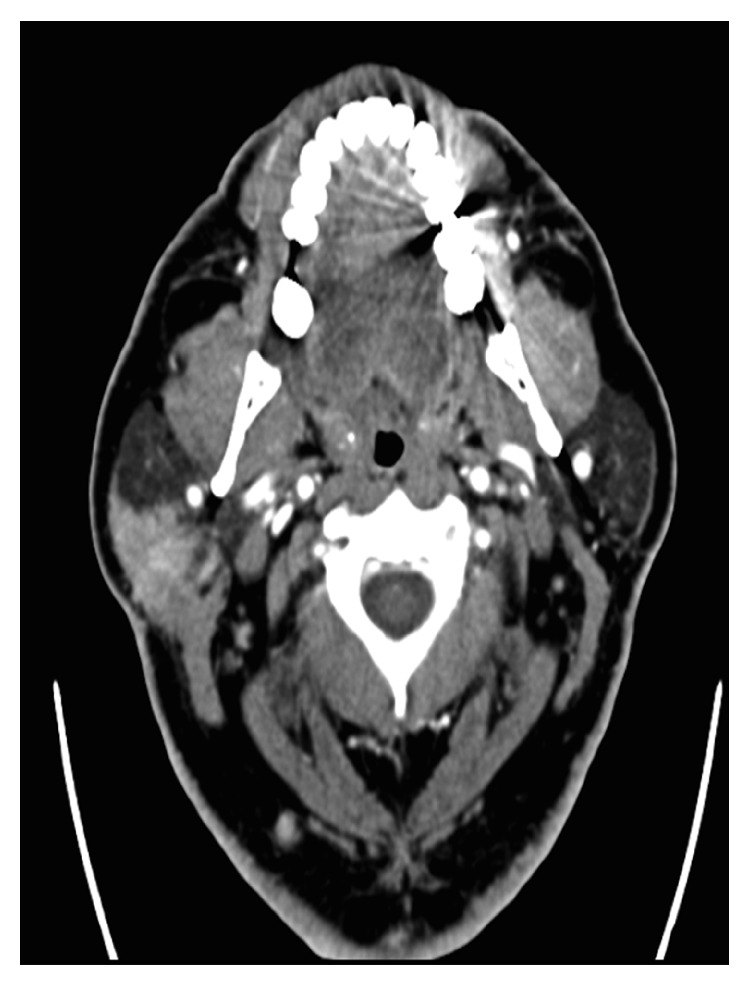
Axial CT with contrast shows a mass in the superficial lobe of the right parotid gland with post-contrast enhancement.
